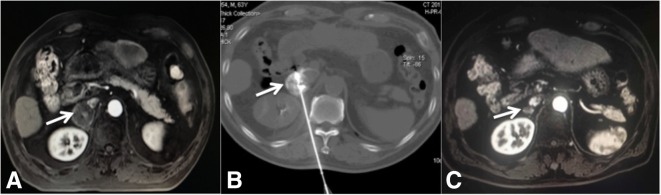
A 63-year-old male patient with right adrenal metastases from HCC who received TACE+RFA. a Pre-operative enhanced MRI, showing right adrenal metastases with uneven enhancement (arrow). b RFA immediately after TACE of the adrenal metastases. c Enhanced MRI 2 years after treatment, showing no enhancement and a smaller tumor (arrow)
